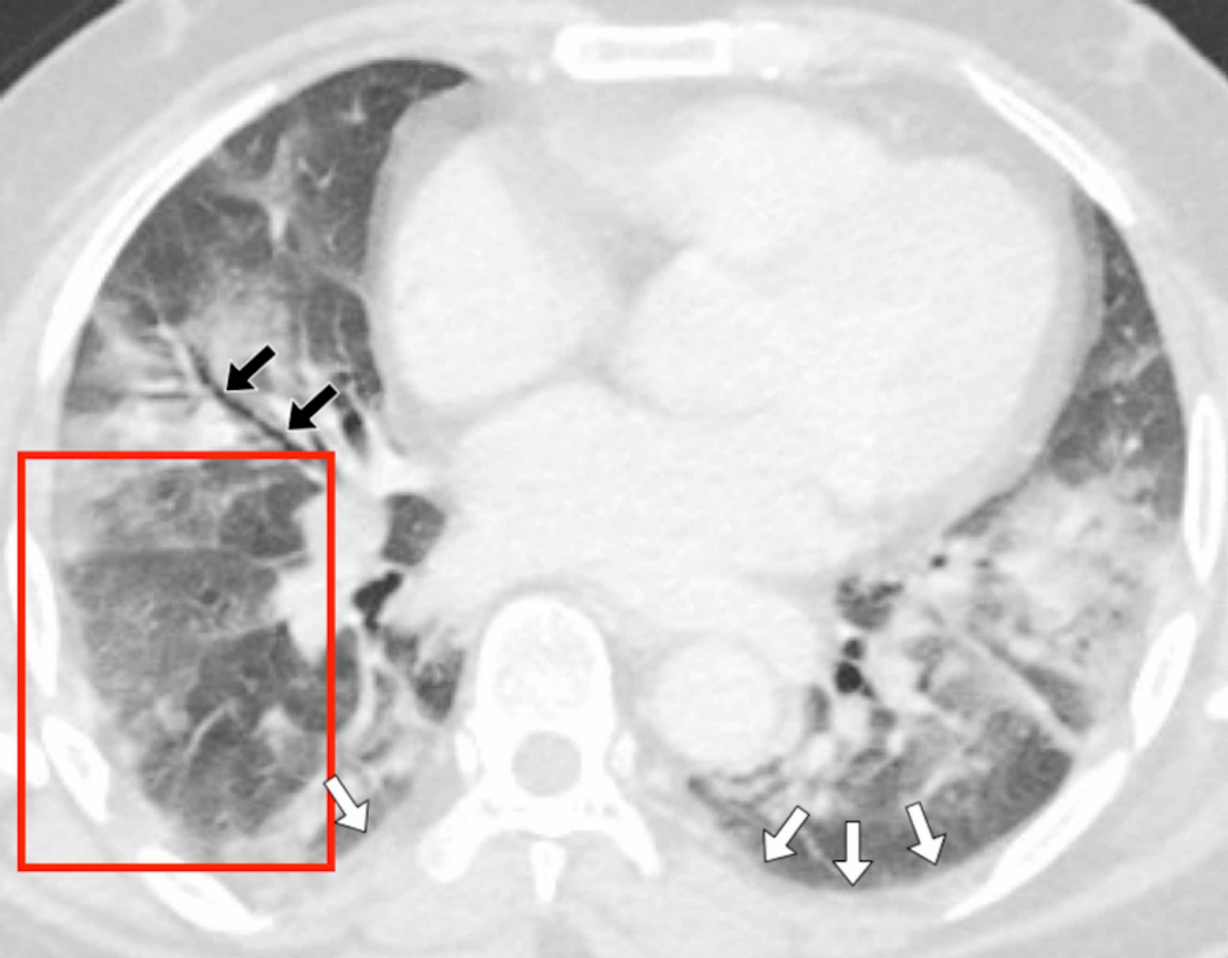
Patient 3 A 53-year-old male patient was admitted to the emergency department with left-sided abdominal pain, decreased appetite, and vomiting. The patient was investigated with a whole-body computed tomography (CT), which demonstrated lung parenchymal changes consistent with COVID-19. (Figure 5a) An axial contrast-enhanced CT of the chest, in lung windows, demonstrates patchy bilateral ground glass and consolidative changes. There is evidence of bi-basal pleural thickening (white arrows), air bronchograms (black arrows), and mosaic attenuation (red box).
